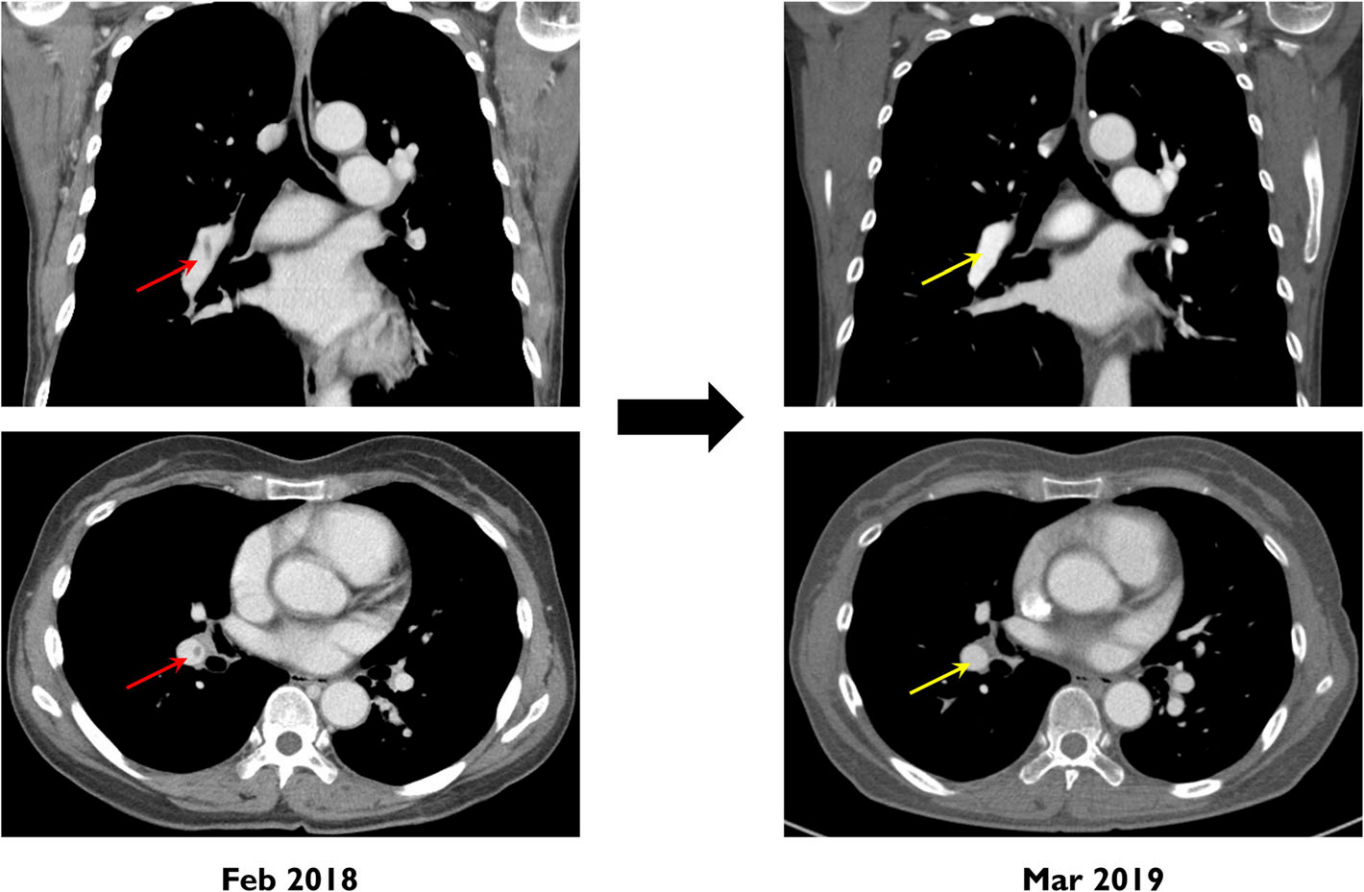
Initial and follow-up chest CT scans. The initial chest CT scan with contrast showed filling defects in right lower segmental pulmonary arteries (left panel, red arrow) and the follow-up CT scan performed 13 months after anticoagulation therapy showed complete resolution of filling defects of right lower segmental pulmonary arteries (right panel, yellow arrow)
